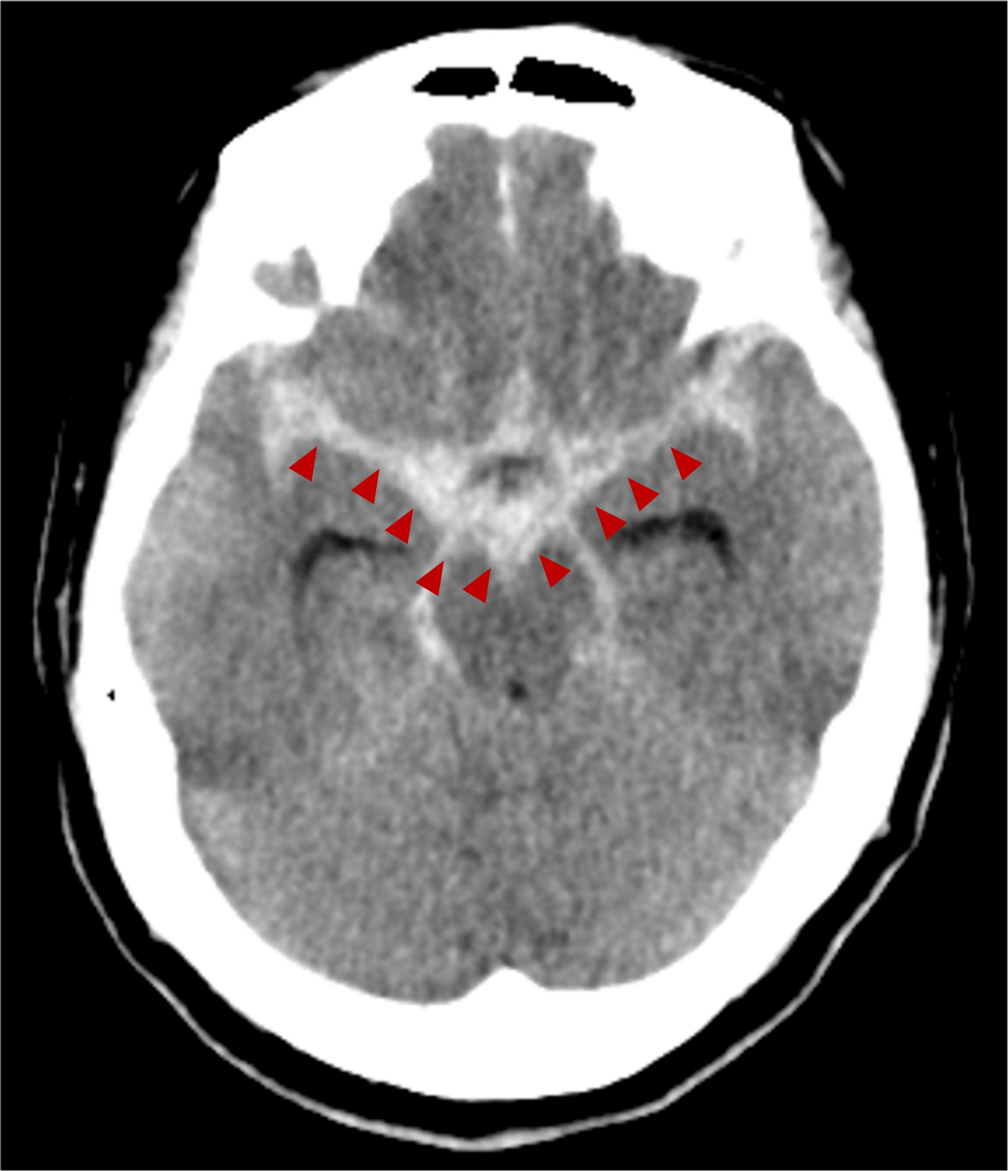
Initial CT Head at admission A thick hematoma was observed in the basal cistern (red arrow head), consistent with a diagnosis of sabarachnoid hemorrhage.
